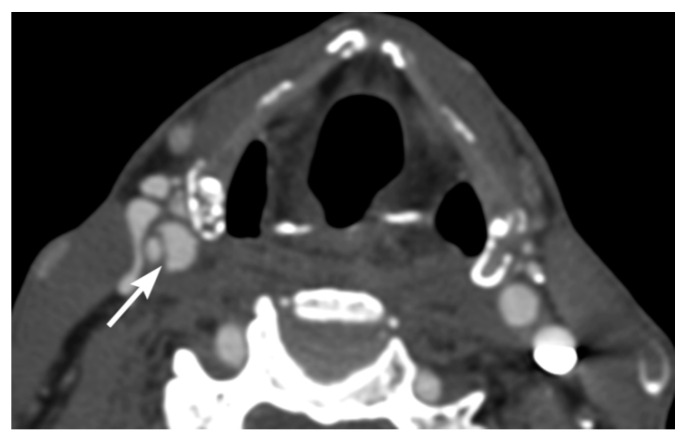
Right common carotid artery injury in a 71-year-old male who presented following a motor vehicle accident. Axial multidetector CT angiographic image shows dissection in the distal right common carotid artery. The located true lumen is moderately narrowed by the false lumen but remains patent.
